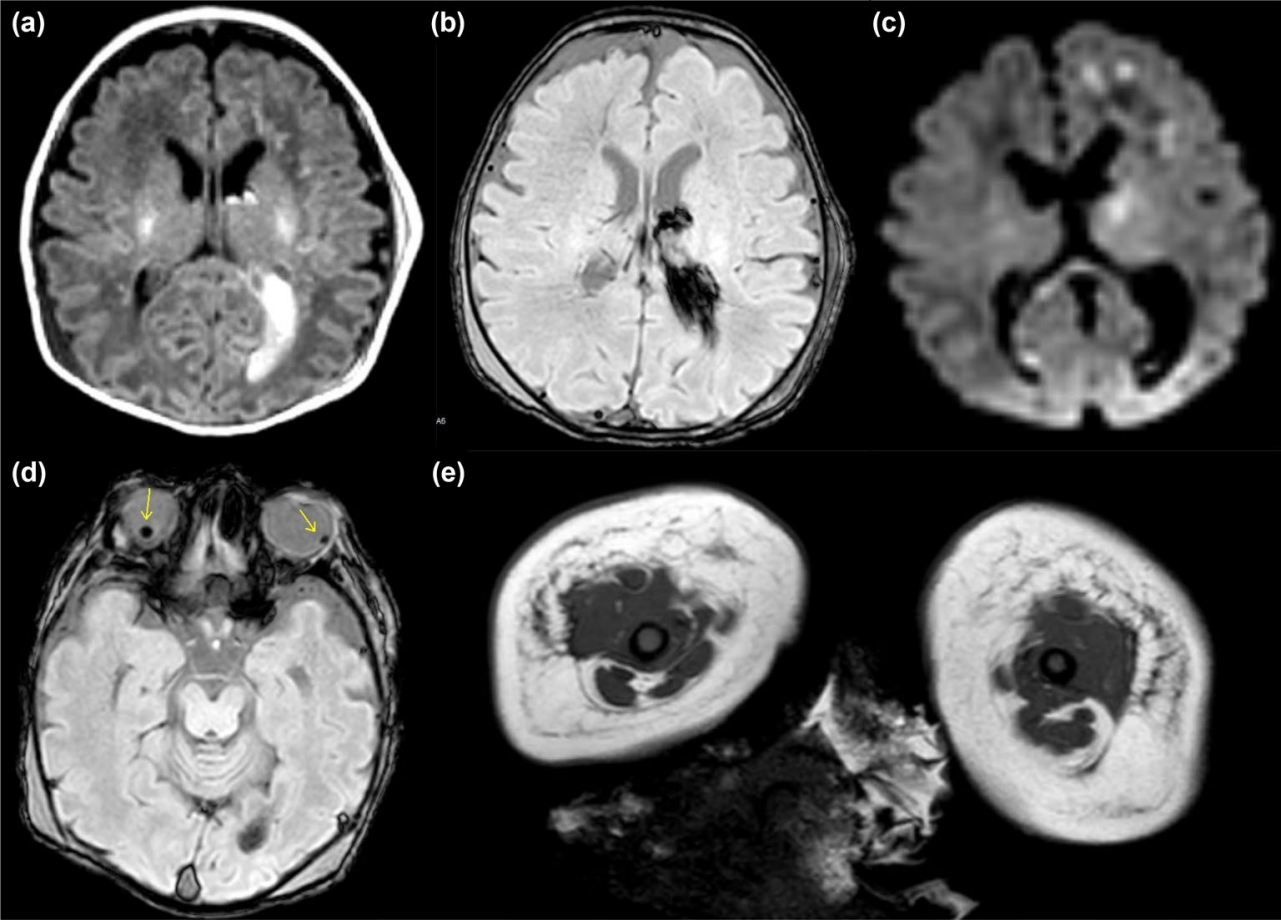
MRI Findings: T1, SWI, and DWI with hemorrhagic infarction (a–c). SWI with retinal hemorrhages (d) and thigh MRI with posterior atrophy (e)
